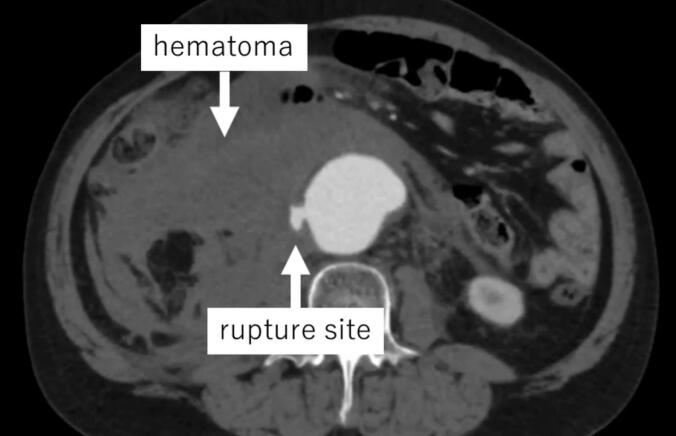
Contrast-enhanced computed tomography revealed a ruptured abdominal aortic aneurysm with a massive right retroperitoneal hematoma.
